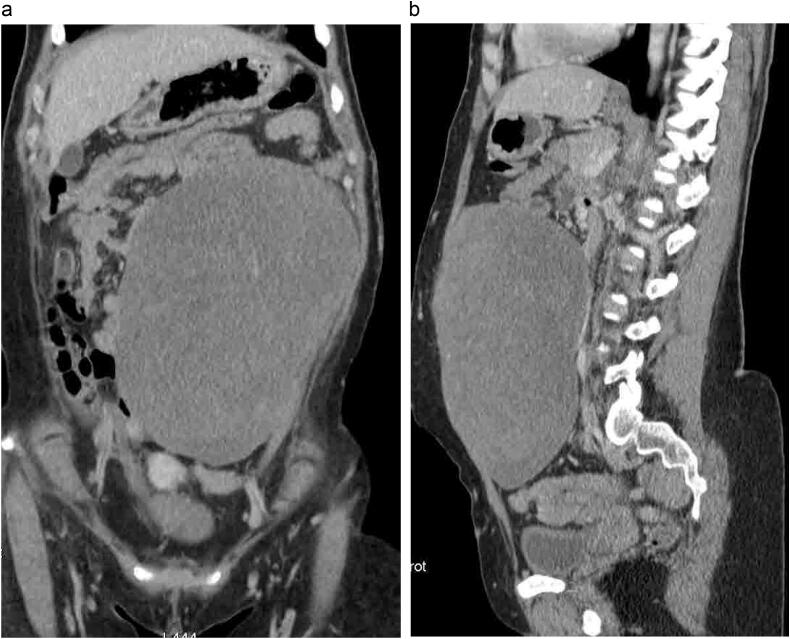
a: The coronal CT scan section shows a well-circumscribed mass on the left side of the abdomen b: The sagittal CT slice reveals the extent of the lesion.
